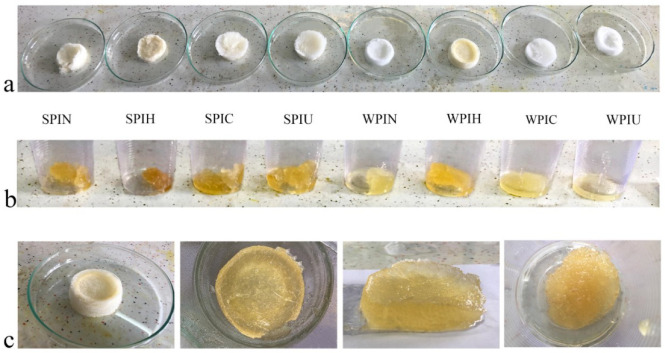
Cryogel and oleogel images resulting from various protein treatments. (a) Cryogels produced from natural and treated protein by foam method. (b) Final oleogel produced from cryogel. (c) Production steps of oleogel from cryogel include oil absorption and homogenization. SPIN: natural soy protein isolate. SPIH: heat-treated soy protein isolate. SPIC: atmospheric cold plasma-treated soy protein isolate. SPIU: ultrasonic-treated soy protein isolate. WPIN: natural whey protein isolate. WPIH: heat-treated whey protein isolate. WPIC: atmospheric cold plasma-treated whey protein isolate. WPIU: ultrasonic-treated whey protein isolate.
